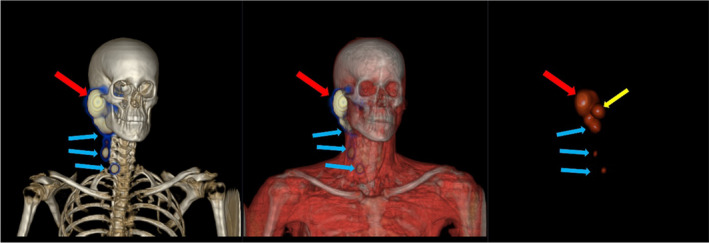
SPECT–CT showing individual lymphatic drainage to SNs from a patient with parotid tumor. Red arrow = injection site around the tumor; blue arrows = SNs in the neck; yellow arrow = intraparotid SN.
